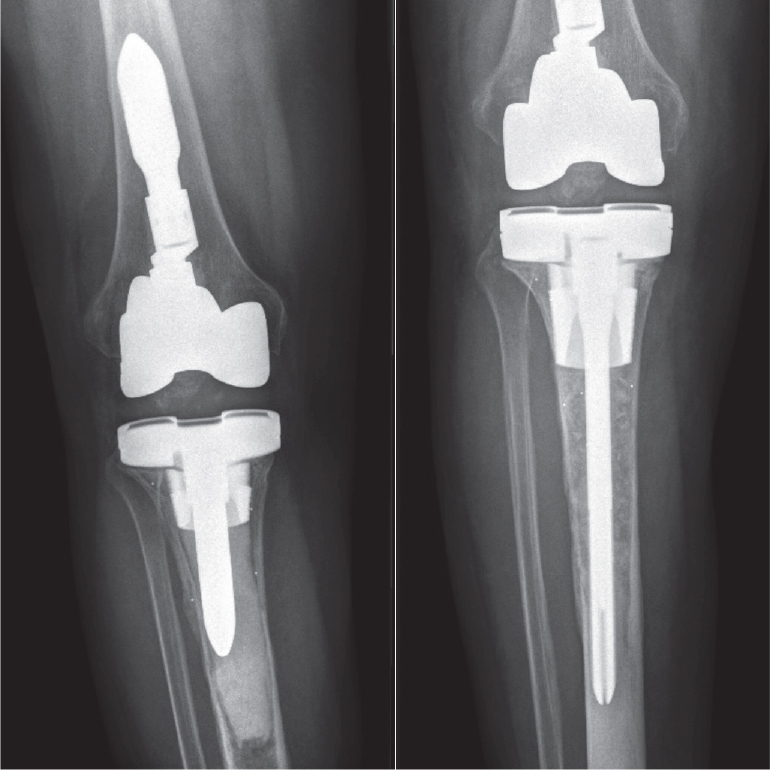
Anteroposterior radiograph of a patient with tibial loosening 14 days before re-revision surgery (left) and 6 weeks post re-revision surgery (right) after intraoperative confirmed tibial loosening. Before the initial revision surgery this patient had moderate epiphyseal and metaphyseal bone loss, mild diaphyseal bone loss, and the initial construct contained a tibial wedge, short cone, and a 120 mm tibial stem.
